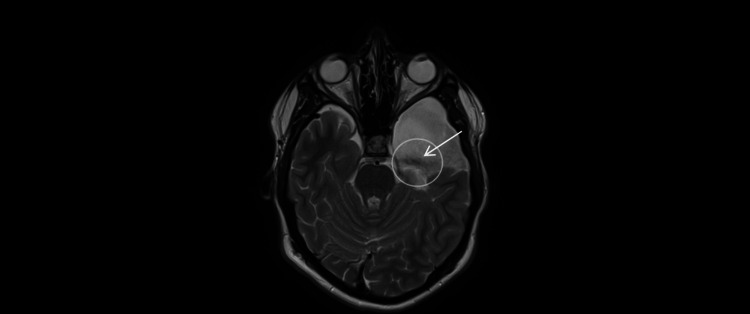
MRI (July 23, 2025; axial T2-weighted; 4-mm slices; 0.5-mm gap). Flow-related artifact along the medial wall of the left middle-fossa arachnoid cyst (arrow, open circle), consistent with persistent patency of the surgical fenestration and cyst-basal cistern communication, in keeping with prior findings.
